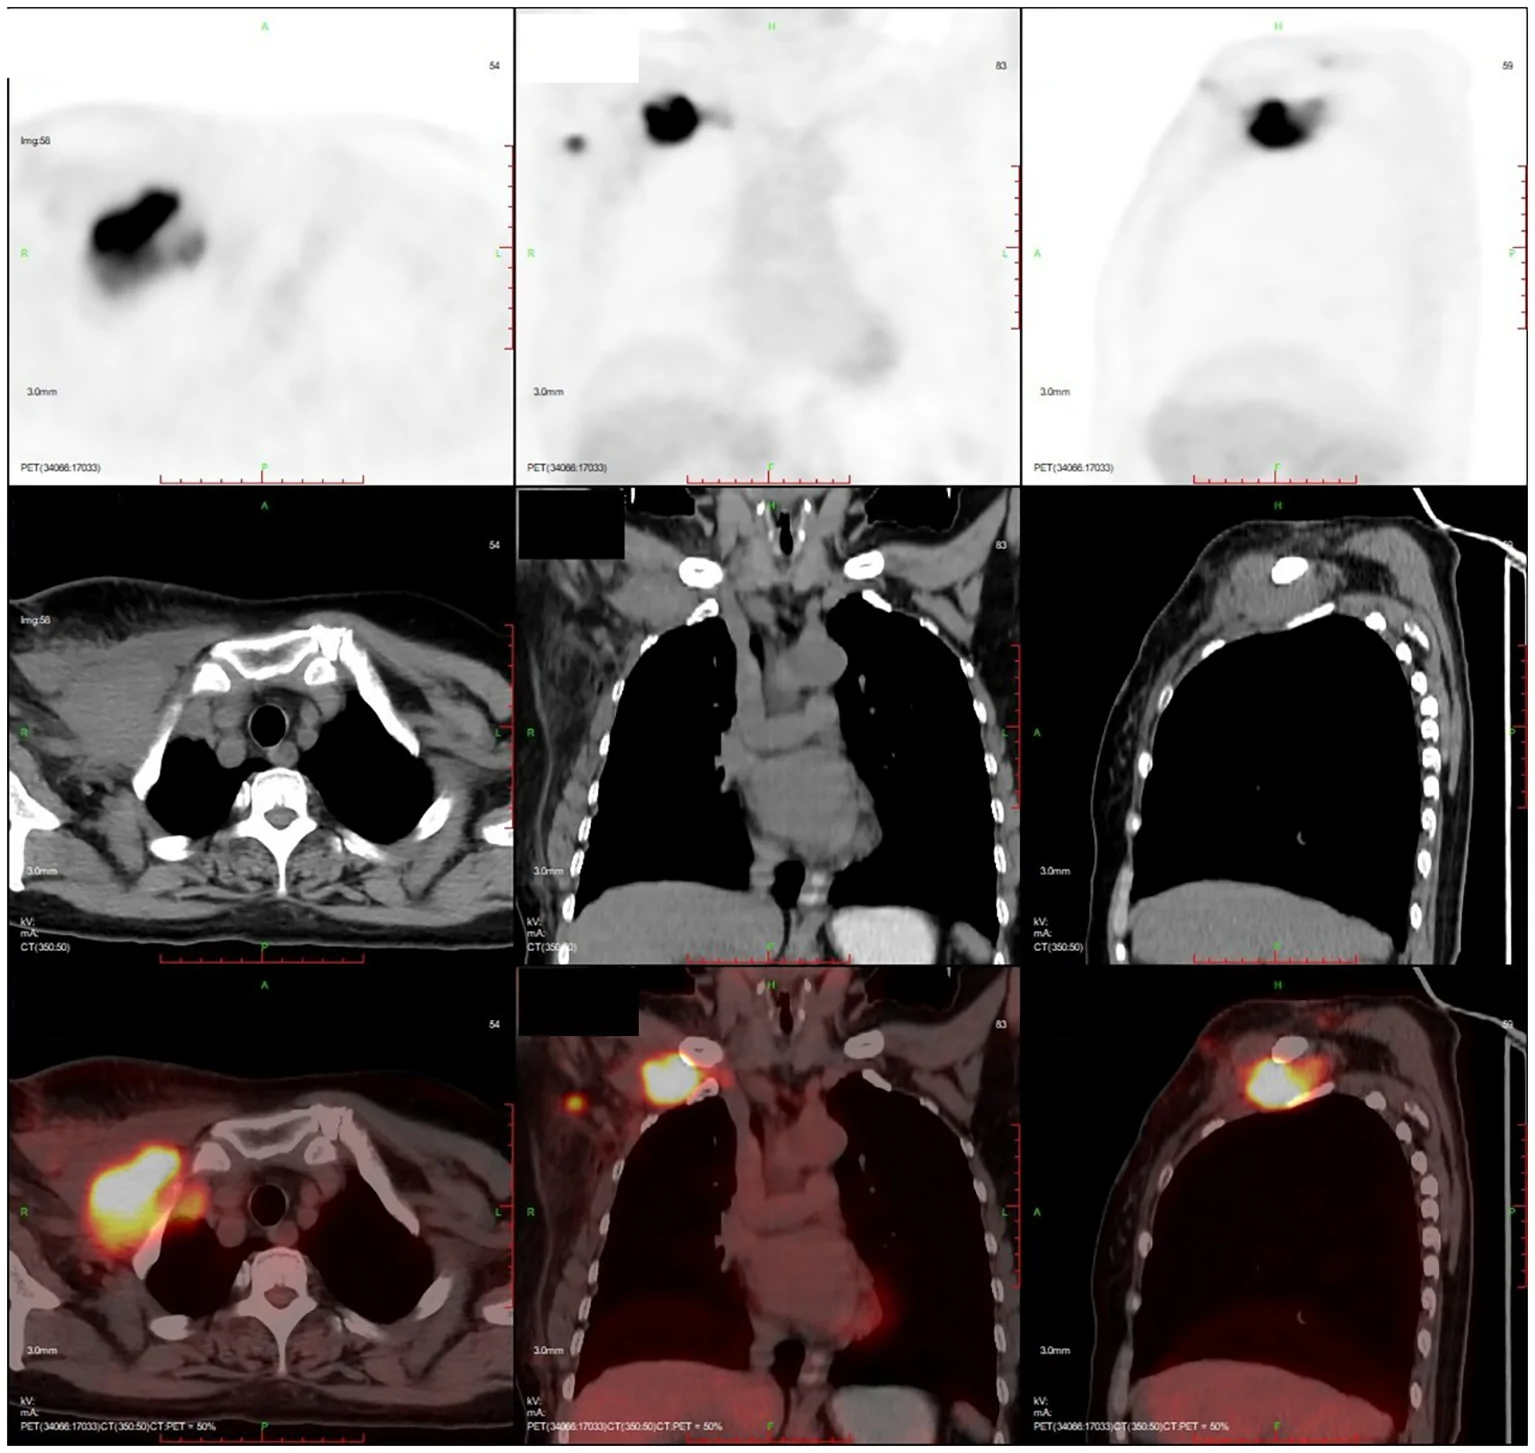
Pre-treatment PET-CT. Maximum-intensity projection and axial images demonstrating hypermetabolic lesions in right clavicular inferior soft-tissue region, right axillary, right supraclavicular and right posterior cervical lymph nodes. SUVmax values up to 21.7, consistent with metastatic disease. No definite primary tumor was identified.
